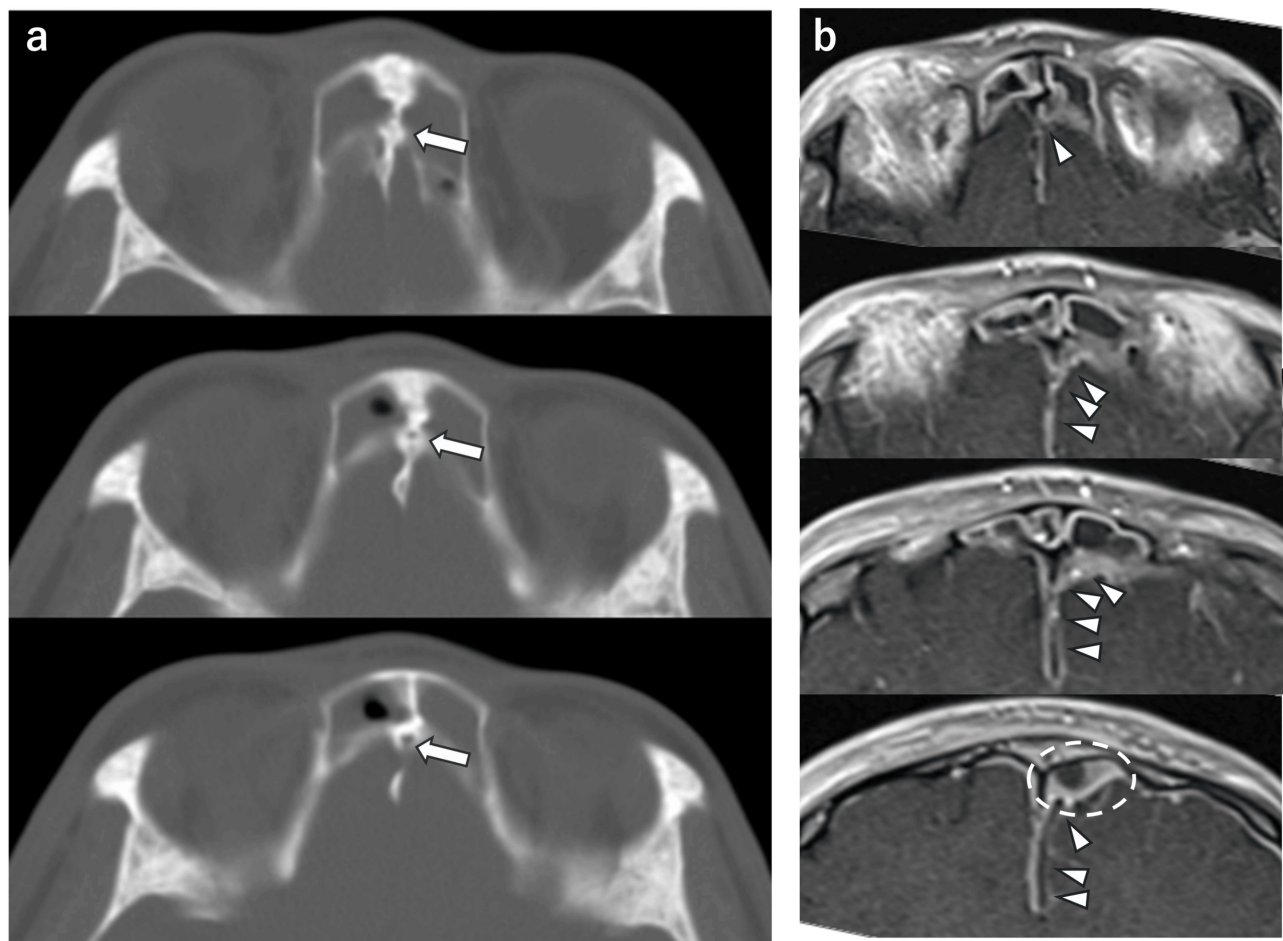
Foramen Cecum a) Axial CT, b) axial MRI T1-weighted gadolinium contrast images. Arrow: foramen cecum; arrowhead: subdural abscess; inside dotted line: subdural abscess CT and MRI T1-weighted gadolinium-enhanced contrast images suggested that an abscess had formed in the epidural region of the left frontal lobe just above the frontal sinus. T1-weighted gadolinium contrast images showed a uniform low-signal area with contrast effect in the epidural region of the left frontal lobe just above the frontal sinus.
